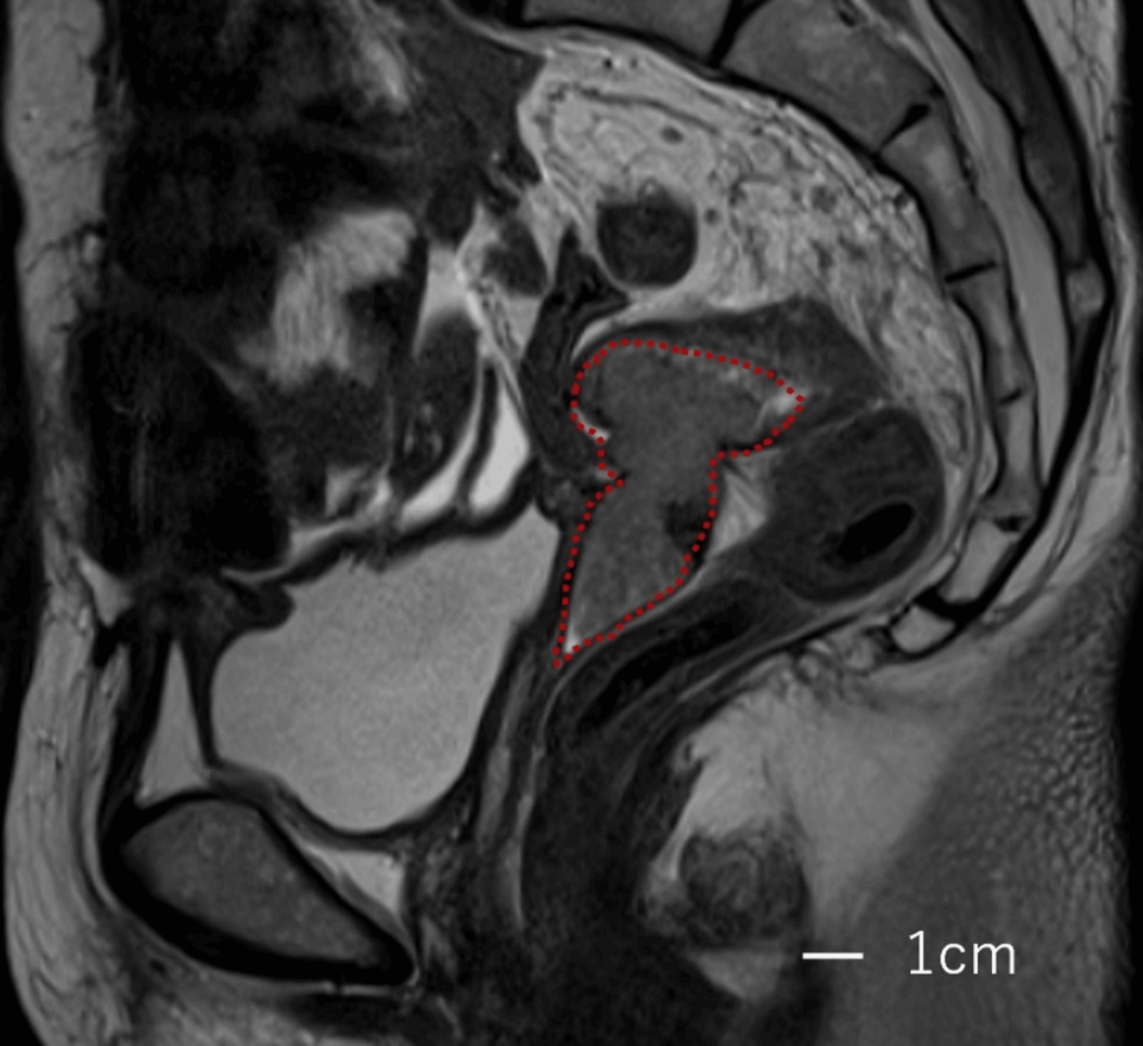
T2-weighted MRI (sagittal), showing the tumor (red dot line) (scale bar = 1 cm) MRI: magnetic resonance imaging
